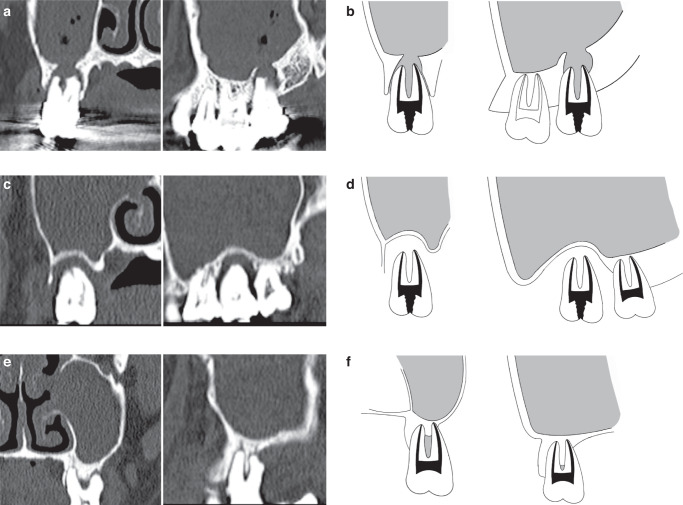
Representative sinus CT images of OMS. a, b Definite evidence: CT images show the discontinuity of maxillary sinus floor in the site of dental lesion. c, d Potential evidence: CT images show a thin layer of floor bone remaining between the maxillary sinus floor and oral lesion. e, f Questionable evidence: CT images show a thick layer of floor bone remaining between the maxillary sinus floor and oral lesion
